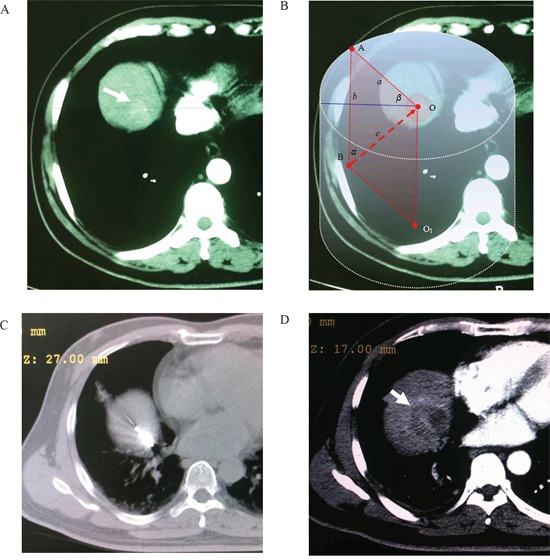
Application of the mathematic puncture model Images obtained in a 55-year-old man with HCC. A. Preoperative transverse CT scan showed An enhanced lesion located in the hepatic dome (arrowhead). B. The maximal area of tumor in the axial plane was selected and a circular delineation was performed. A cylinder model was established after selecting the center of tumor's maximal circle in axial plane as its top surface center (point “O”). A vertical plane which crossed point “O” and “A” (the skin poin on the circle of cylinder's top surface) was determined (β = 45°). According to the calculation method of this model, the puncture route was determined as “BO” after selection of puncture site “B”. C. A MW antenna was percutaneously inserted into the lesion from the bottom up along the puncture route caculated by the established model (α = 45°, *d* = 11.3 cm). D. Arrow indicated complete necrosis of tumor without enhancement after ablation.
